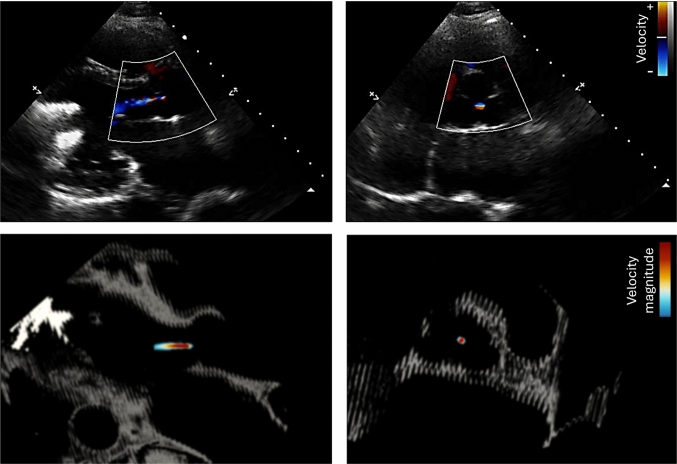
Color Doppler echocardiography images (top row) showing two different views of the aortic valve regurgitant jet. The Doppler flow is colored based on magnitude and direction, with flow moving toward the probe as positive and the flow moving away from the probe as negative. Corresponding computational fluid dynamics (CFD) solutions are shown in the bottom row, aligned to the same views. Here, the flow is colored only by the velocity magnitude. The CFD results are overlaid on the patient’s CT-derived anatomy to visualize the surrounding cardiac structures
